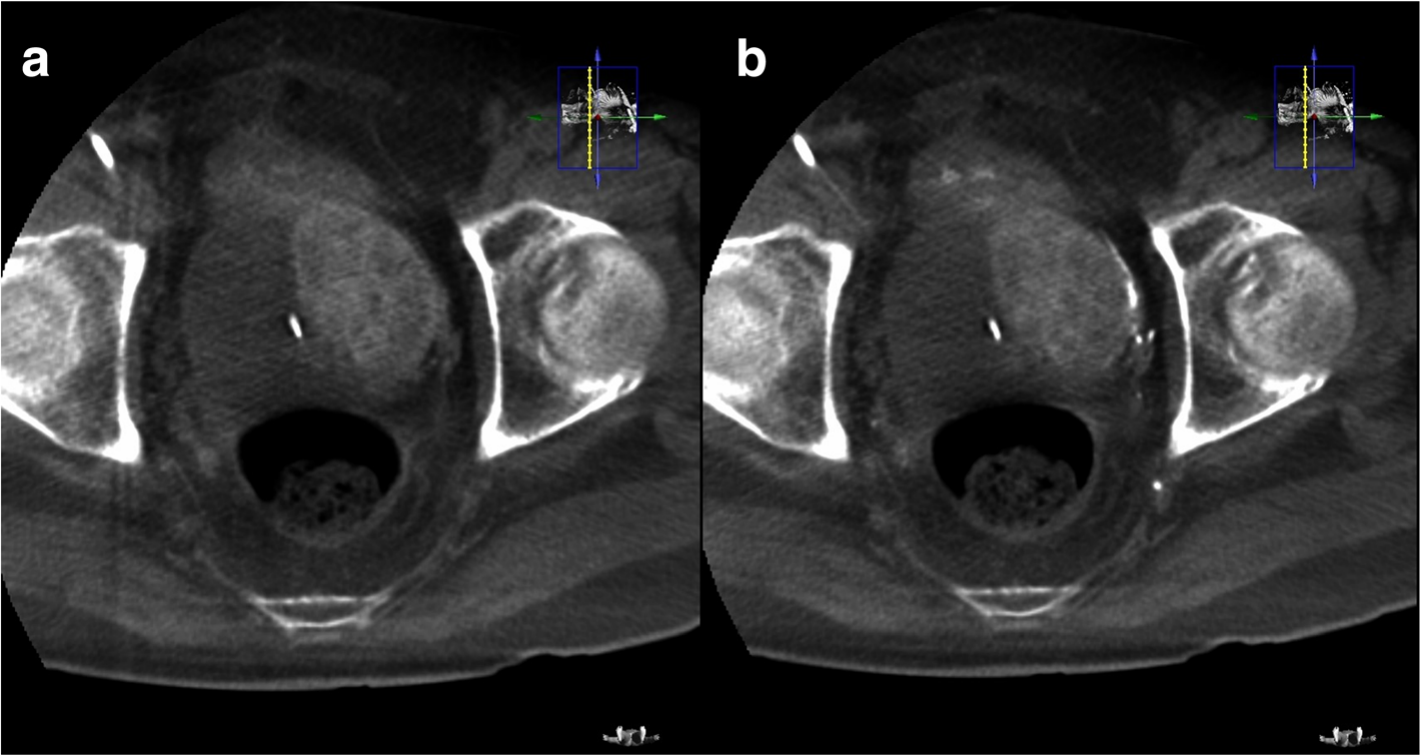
a Non-contrast CBCT after embolization of the LPA shows retention of contrast medium in the left aspect of the prostatic parenchyma without filling defect. Some contrast medium was excreted from the kidneys and accumulated in the urinary bladder. b Contrast CBCT after embolization of the LPA shows no further enhancement of the left aspect of the prostatic parenchyma, suggesting adequate embolization
